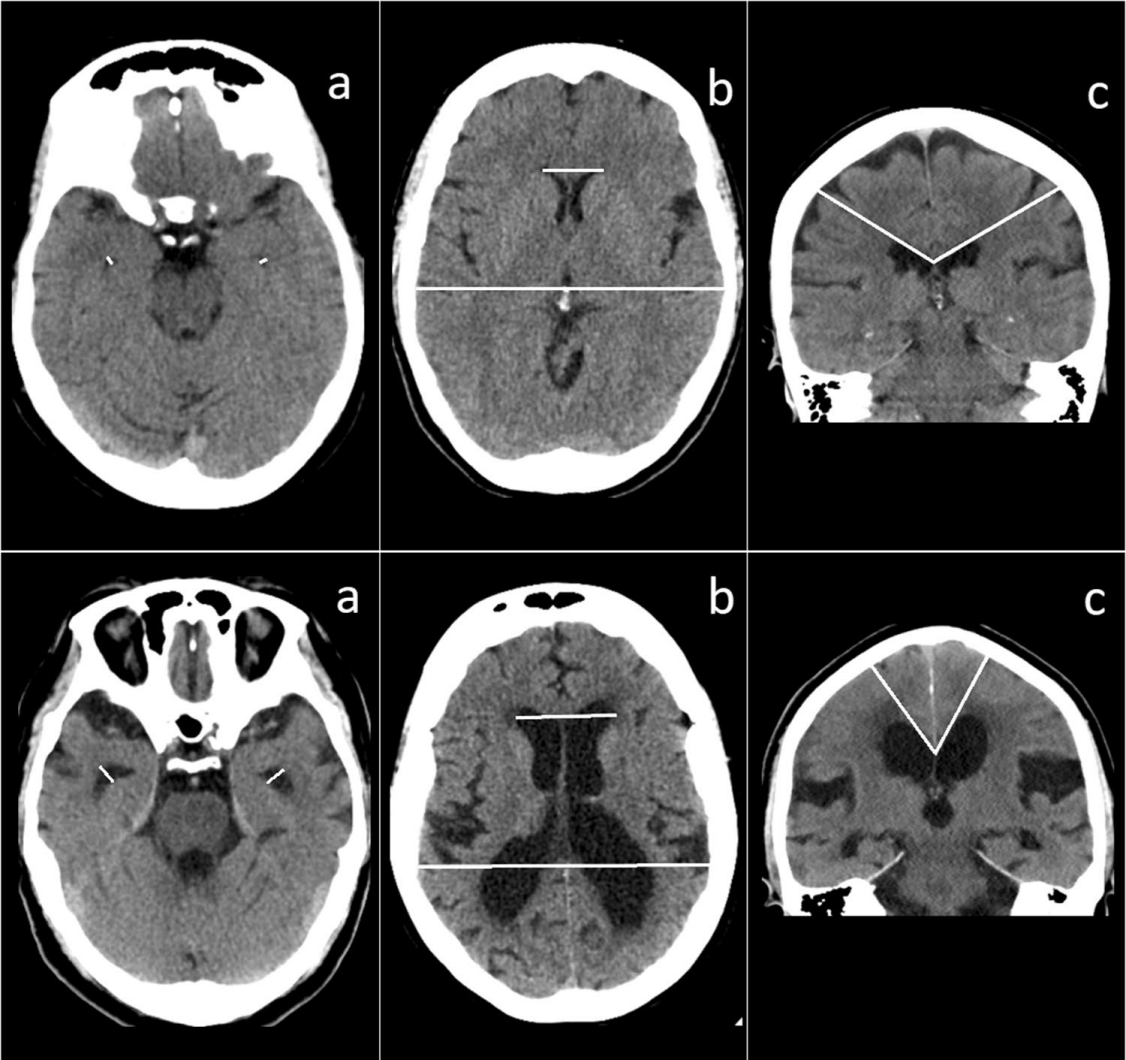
Radiological measurements. The first row shows CT-samples from healthy individuals. The second row represents symptomatic samples. NOTE: Measuring lines in white; (a) width of the temporal horns; (b) Evans’ index, and (c); the callosal angle.
